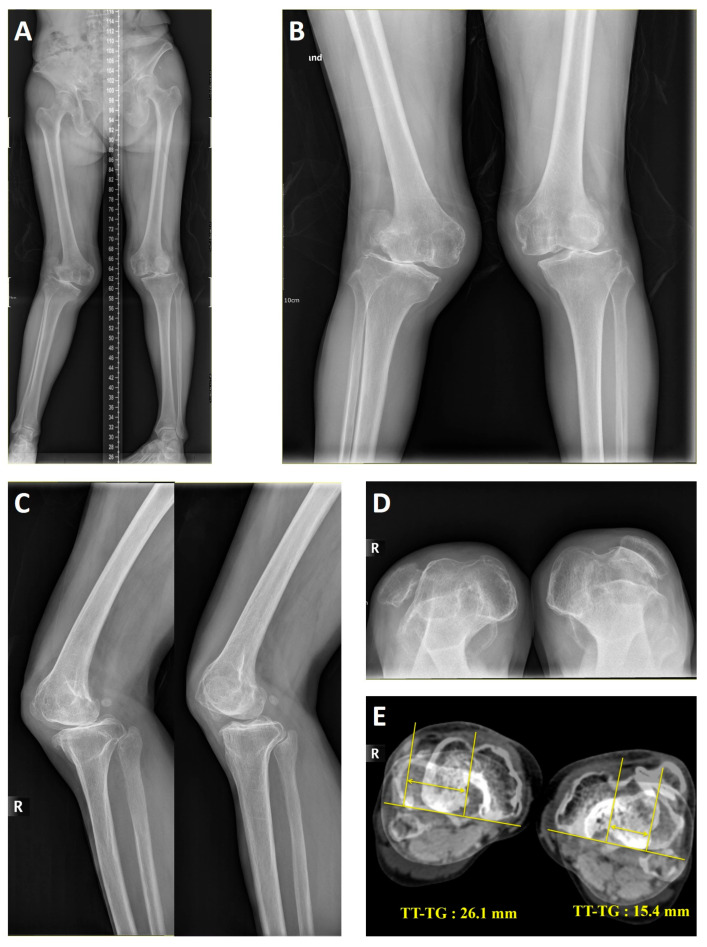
(A–E). Bilateral secondary osteoarthritis with neglected dislocation of the patella in a 68-year-old female patient (Cases No. 1 and No. 2): (A) long-leg standing anteroposterior view; (B) standing anteroposterior view; (C) lateral view of right and left knees; (D) skyline view; and (E) rotational computed tomography image showing the tibial tuberosity–trochlear groove (TT-TG) distance.
